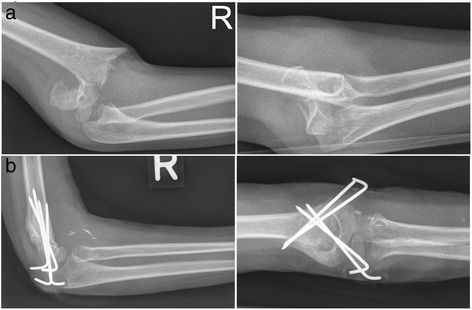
Radiographs in two planes: a preoperative and b postoperative after 6 weeks
